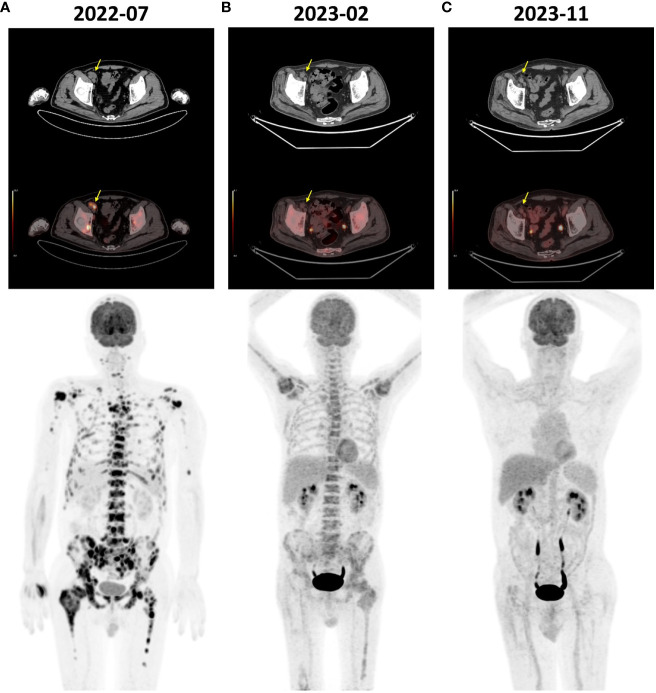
FDG-PET/CT images of the patient. (A) The pre-treatment scan (July 2022) revealed diffuse bone FDG uptake (SUVmax 15.6) and multiple lymph node enlargements adjacent to the right iliac vessels (yellow arrow) showing increased FDG uptake (SUVmax 13.8). (B) The scan before the 5th cycle of treatment (February 2023) revealed a significant reduction in bone FDG uptake (SUVmax 5.3) and regression of the enlarged lymph nodes (yellow arrow). (C) The scan conducted at the 14th month post-treatment initiation (November 2023) showed normal systemic bone FDG uptake and no evidence of disease. FDG, fluorine-18 fluorodeoxyglucose; PET/CT, positron emission tomography/computed tomography; SUVmax, maximum standardized uptake value.
